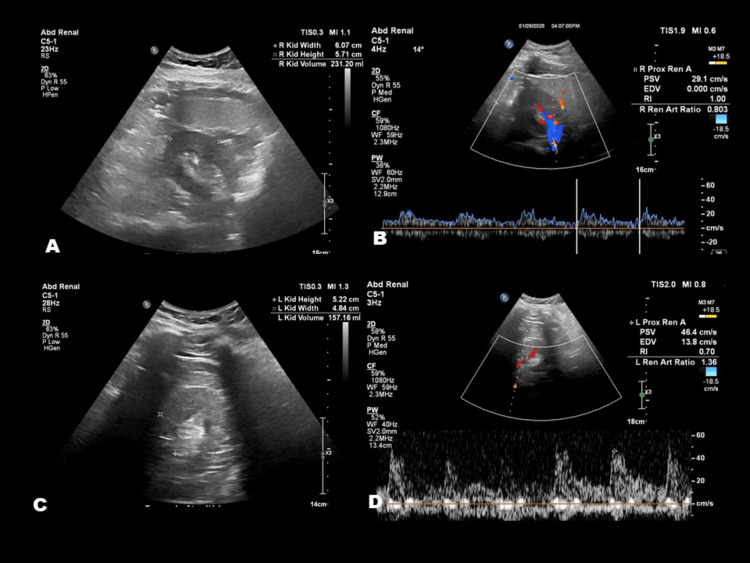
Bilateral renal ultrasound. Bilateral renal ultrasound demonstrates increased echogenicity of the renal cortices, consistent with medical renal disease (A: right; C: left). No evidence of renal artery stenosis is noted bilaterally (B: right; D: left).
